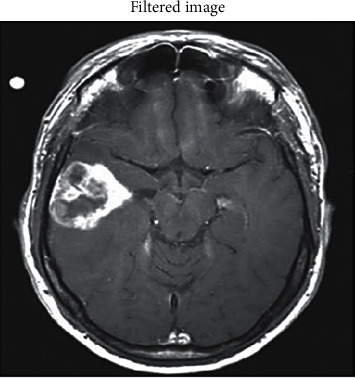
Filtered input MRI image.
